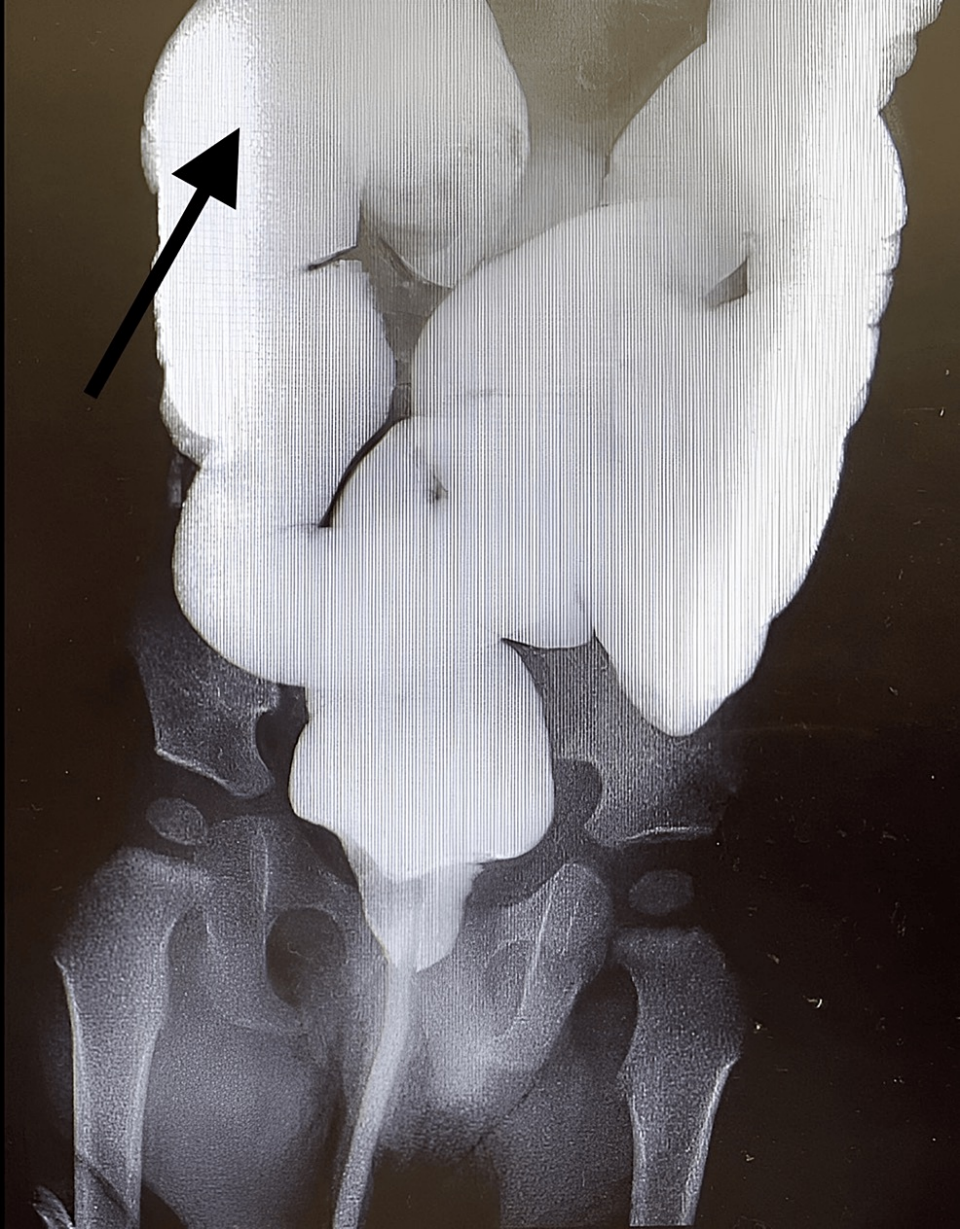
Day 1 X-ray gastrografin enema image showing the reduced obstruction
